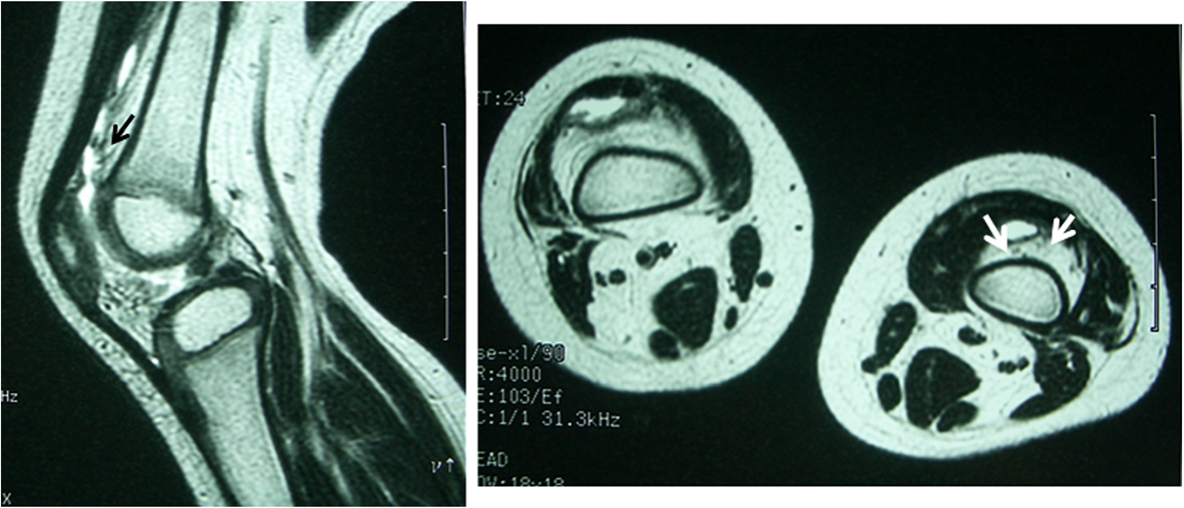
Magnetic resonance imaging (T2-weighted) at 2 years showed a small mass in her knee joint (arrows).
